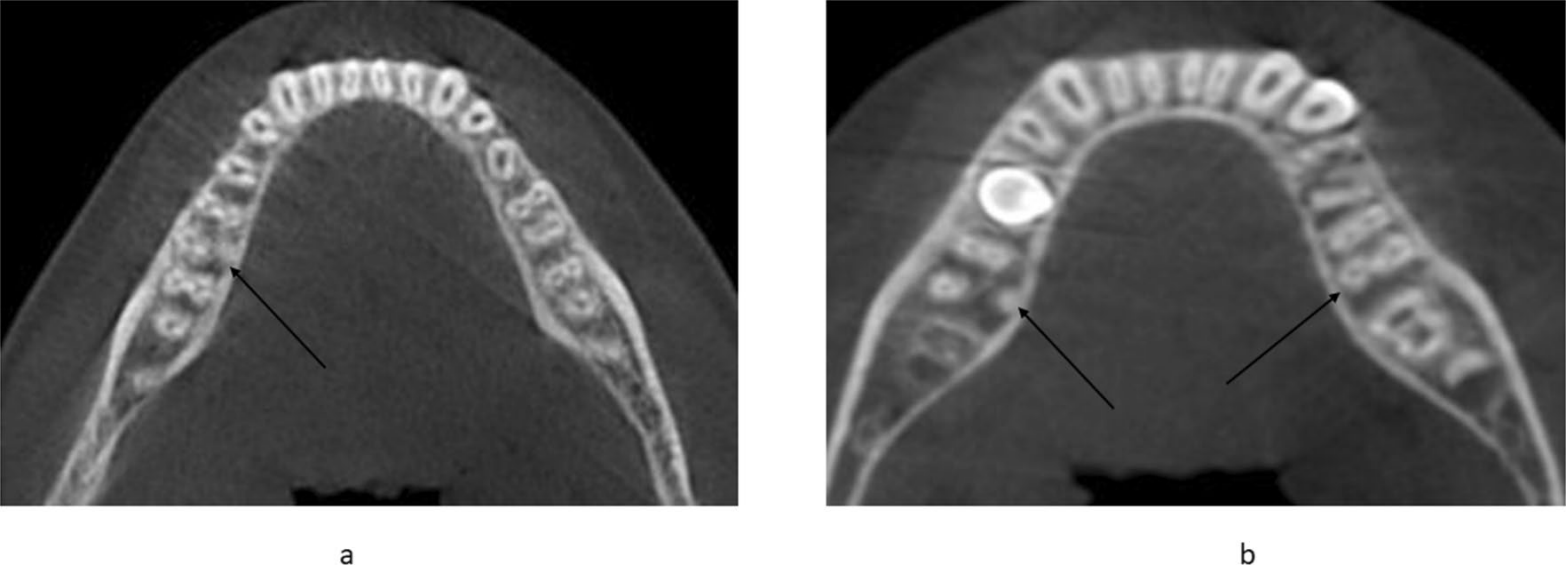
CBCT images showing (a) Unilateral and (b) Bilateral distribution of DLR in MFMs.
